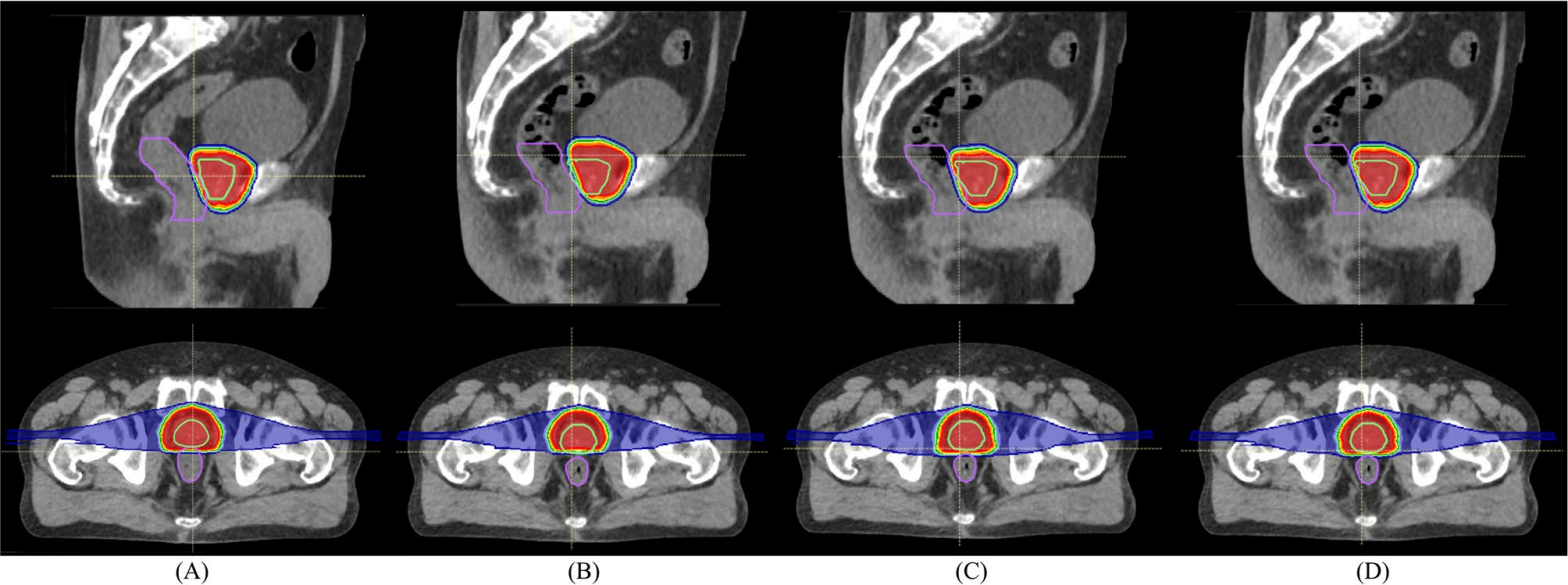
Dose distributions (case no.7) on (A) the planning CT and on the treatment CT (CT1) with (B) intensity-based image registration, (C) target-based image registration, and (D) WEPL-based image registration. Light green and purple lines outline the CTV and rectum, respectively.
